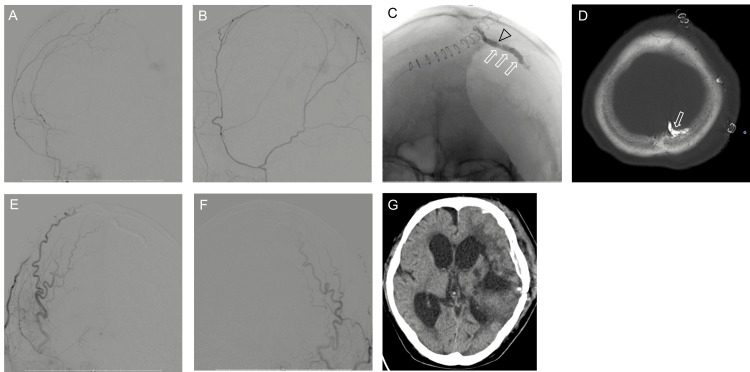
Anteroposterior (a) and lateral (b) views of the right middle meningeal artery angiogram showing the access route to the shunt pouch. Craniography (c) and computed tomography (d) showing the tip of the microcatheter in the venous pouch (open arrowhead) and the cast of N-butyl-2-cyanoacrylate (open arrow). Anteroposterior view of the right (e) and left (f) external carotid artery angiograms after treatment demonstrating no arteriovenous shunt. Follow-up computed tomography (g) showing no rebleeding
